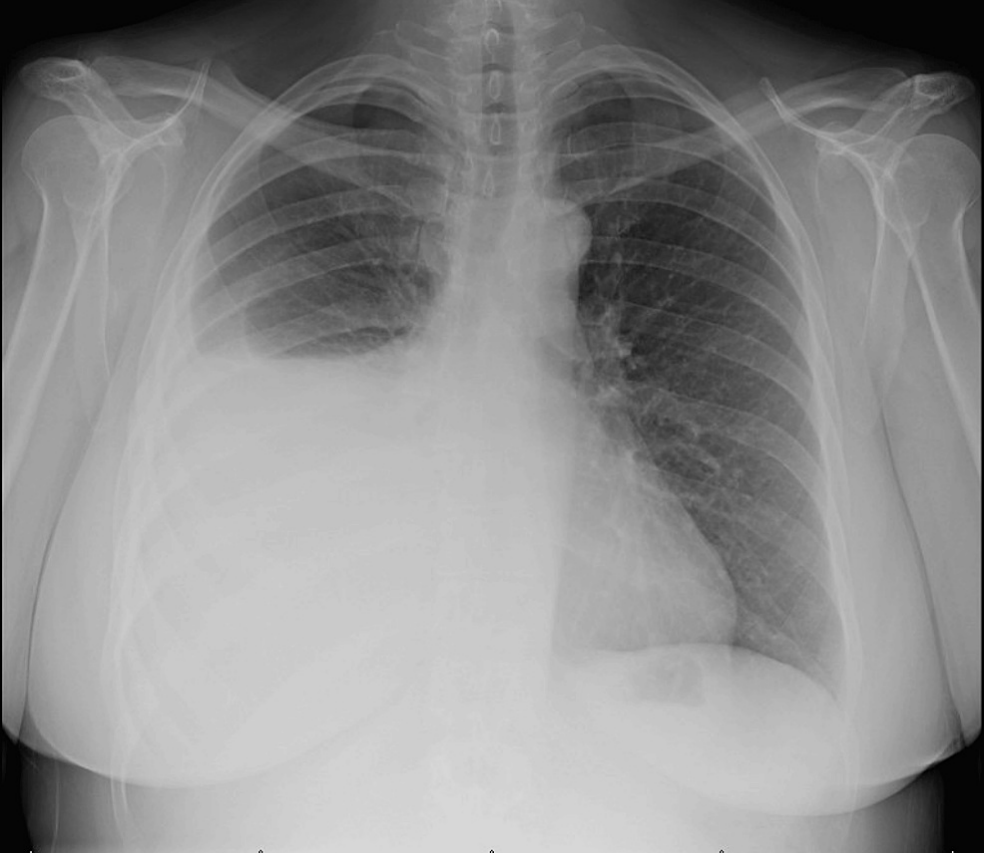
The patient's chest X-ray showing moderate to large right pleural effusion. The cardiomediastinal silhouette is within normal limits. No overt pulmonary congestion.
